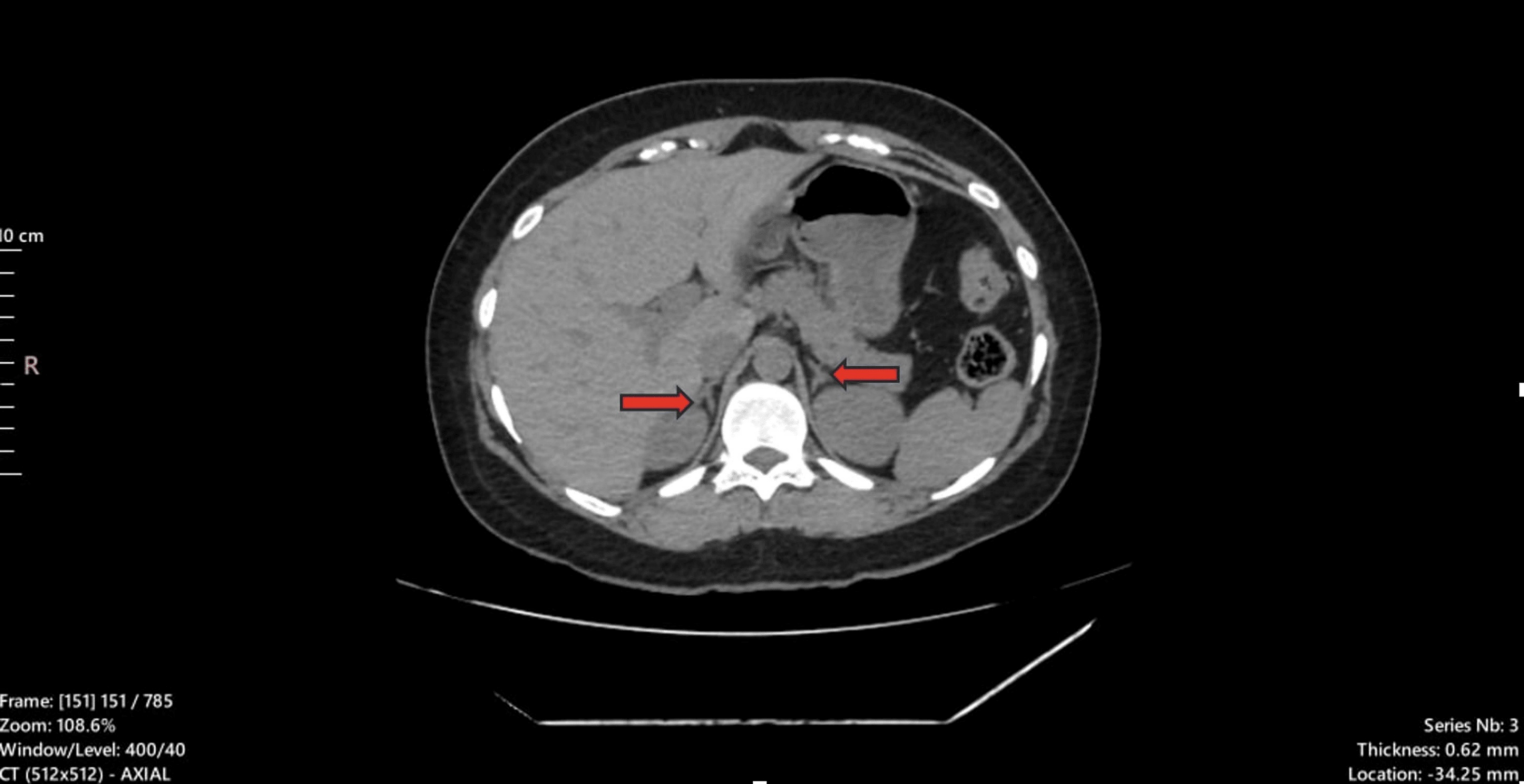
CT scan of the abdomen of the patient (axial view) showing no radiographic abnormality in the adrenal glands bilaterally, as marked by red-colored arrows. Note: The image was evaluated by a professional radiologist.
